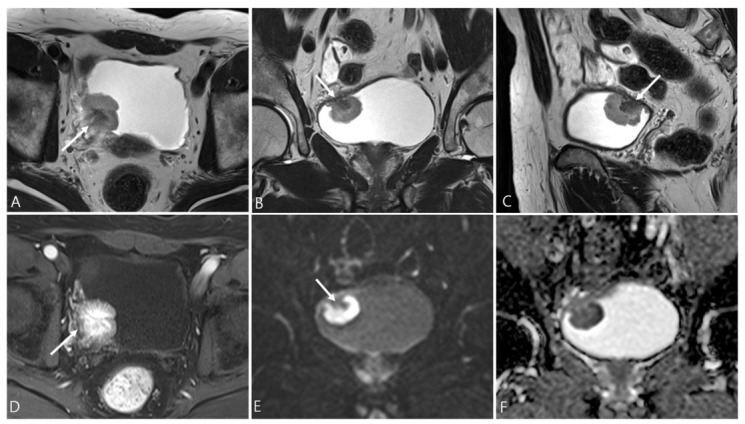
VI-RADS 2 or 3—Limitations of VI-RADS scoring: muscle invasion in a tumor with an apparent stalk at the bladder dome. A 69-year-old male presenting with a papillary mass at the right posterior dome of the bladder. (A) Axial T2-weighted imaging (T2WI) demonstrates a papillary mass at the right posterior dome; owing to the unfavorable anatomical location and suspected partial volume artifact, the accurate assessment of the tumor stalk and muscularis propria integrity was limited, leading to an equivocal VI-RADS 2 or 3 assessment on axial imaging alone. (B,C) Coronal and sagittal T2WI clearly delineate the tumor stalk sign with an intact low-signal muscularis propria; based on these multiplanar findings, the lesion was evaluated as VI-RADS 2. (D) Axial T1-weighted contrast-enhanced imaging (T1CE) shows early mucosal enhancement; however, evaluation of the inner layer and stalk remained suboptimal owing to the dome location. (E) Coronal diffusion-weighted imaging (DWI; b = 1000 s/mm^2^) demonstrates marked diffusion restriction within the tumor lesion, sparing the tumor stalk, which is suggestive of the inchworm sign. without evidence of extension into the muscularis propria. (F) Coronal apparent diffusion coefficient (ADC) map confirms significantly reduced ADC values within the lesion. Based on multiplanar mpMRI assessment, the overall imaging features were interpreted as VI-RADS 2, primarily guided by the tumor stalk sign on coronal and sagittal T2WI and the inchworm sign on DWI demonstrating an intact muscularis propria. However, subsequent TURBT unexpectedly confirmed muscle-invasive urothelial carcinoma. This case highlights a well-recognized pitfall of VI-RADS scoring: Kim et al. reported that approximately 11% of bladder tumors demonstrating a visible stalk on MRI (corresponding to a positive predictive value of 89% for the stalk sign) were ultimately confirmed as muscle-invasive on pathology [41], underscoring the inherent limitations of morphological assessment even with multiplanar imaging. Furthermore, although coronal and sagittal imaging are invaluable for dome lesions, they may paradoxically contribute to understaging when a partial volume artifact mimics an intact muscularis propria. Following neoadjuvant chemotherapy (NAC), partial cystectomy was performed. Preoperative mpMRI showed no evidence of residual tumor, and the final pathological staging confirmed a complete response (ypT0).
